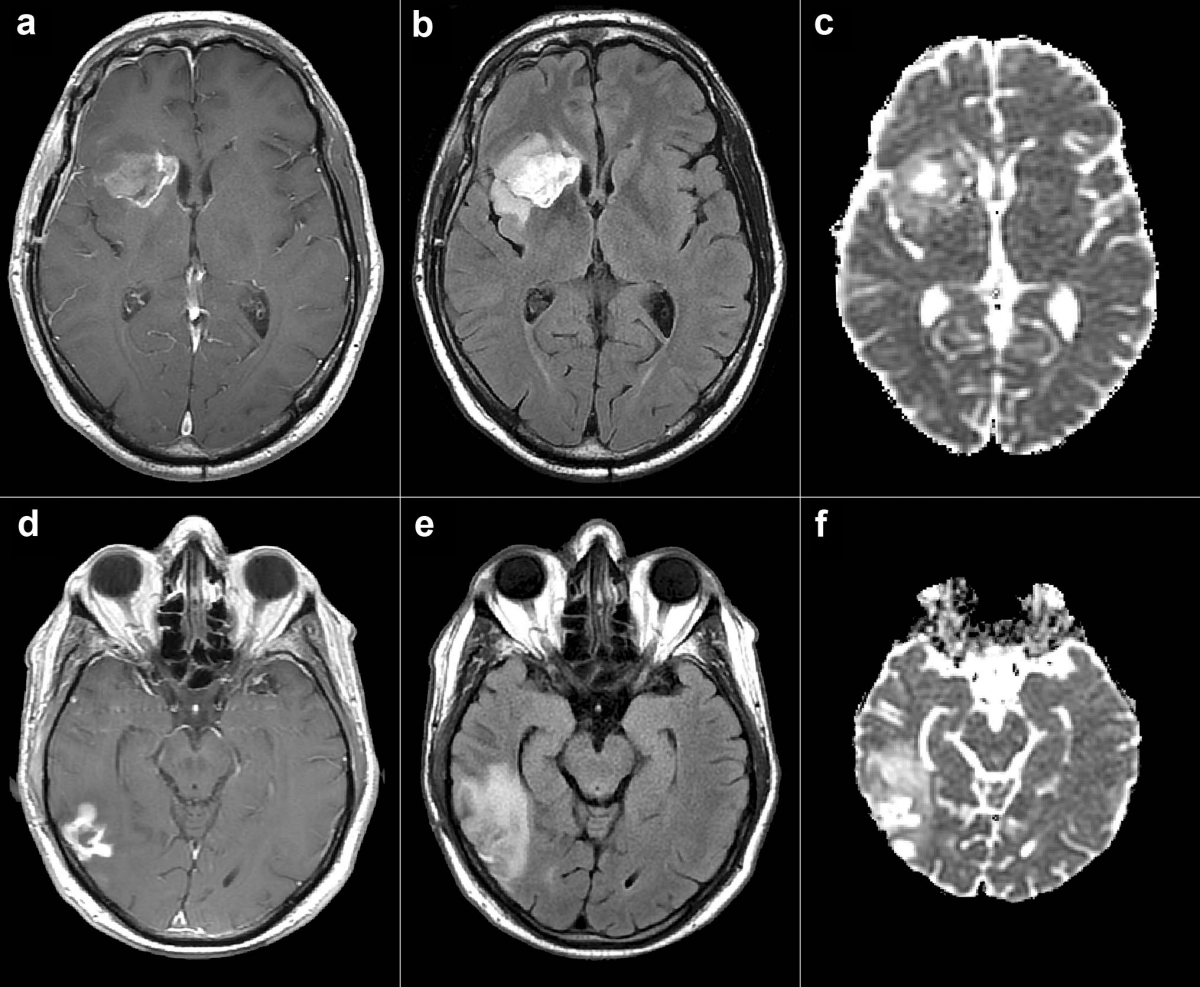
Initial MR images in two subjects. Top row shows initial T1 SE post contrast (a), FLAIR (b) and ADC (c) in one subject that achieved PFS of 29.17 months and OS of 56.5 months. Bottom row shows initial T1 SE post contrast (a), FLAIR (b) and ADC (c) in one subject that achieved PFS of 3.67 months and OS of 12.67 months. *SE* spin echo, *FLAIR* fluid-attenuated inversion recovery, *ADC* apparent diffusion coefficient, *PFS* progression-free survival, *OS* overall survival
